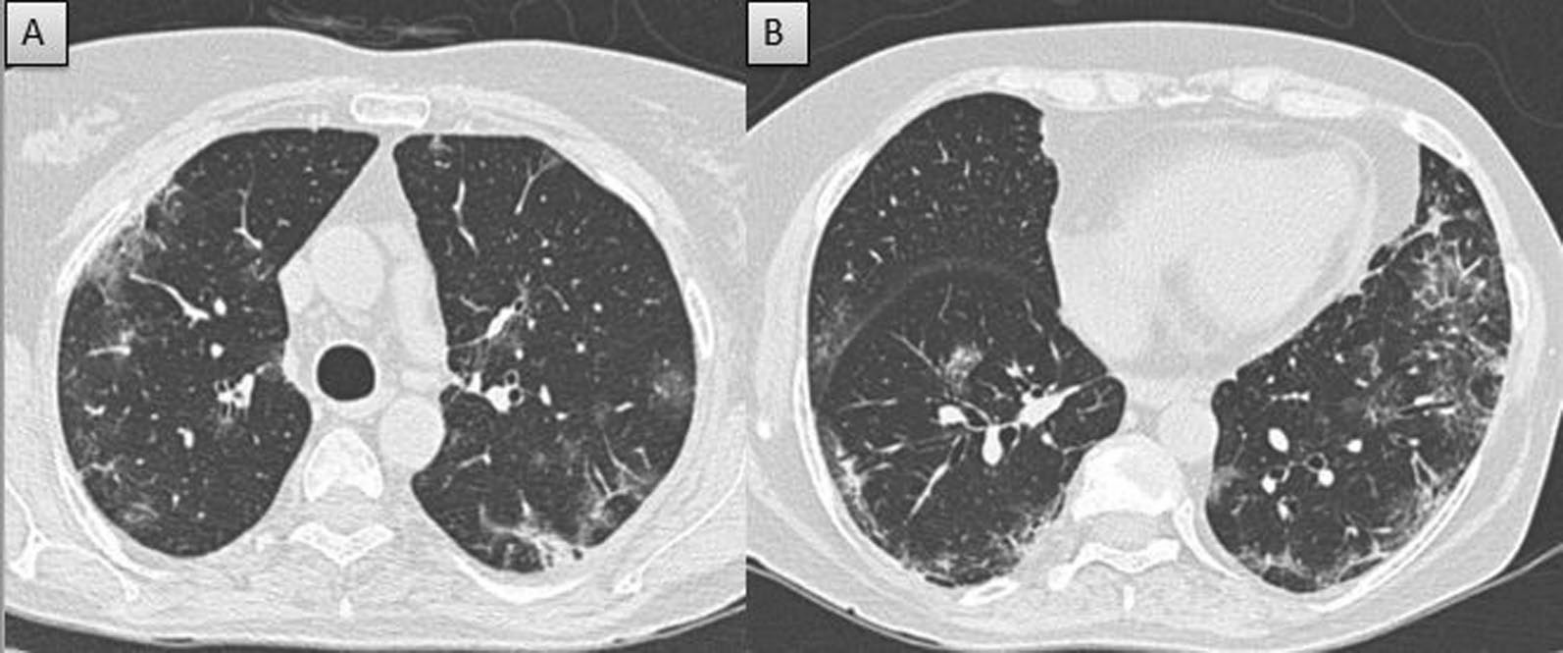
Moderate severity in partially vaccinated patient: A and B Axial CT thorax (lung window) of a 61-year-old COVID pneumonia patient who had received single dose of Covid vaccine shows diffuse peripheral patchy ground glass opacities and interstitial thickening with CT severity score of 14/25 (moderate severity)
